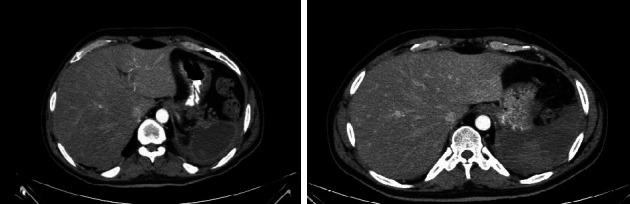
Abdominal CT shows a large collection of liquid on the left subdiaphragmatic space that contains solid material and is encapsulated.
